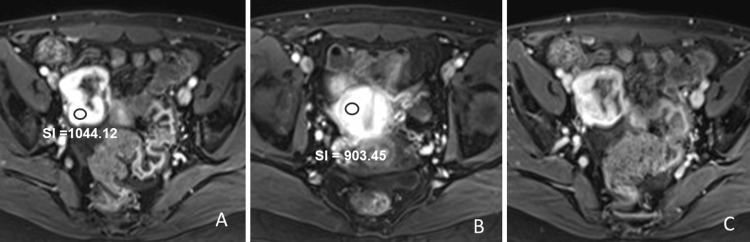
Non-dynamic contrast-enhanced T1-weighted images (A, B) 30 seconds after the gadolinium injection, and (C) 120 seconds after the gadolinium injection. On non-dynamic contrast-enhanced T1-weighted images, the adnexal mass (A, signal intensity = 1044.12) is enhanced more intensely than the myometrium (B, signal intensity = 903.45) 30 seconds after the gadolinium injection. The mass is enhanced with an internal poorly enhanced area 120 seconds after the gadolinium injection (C).
